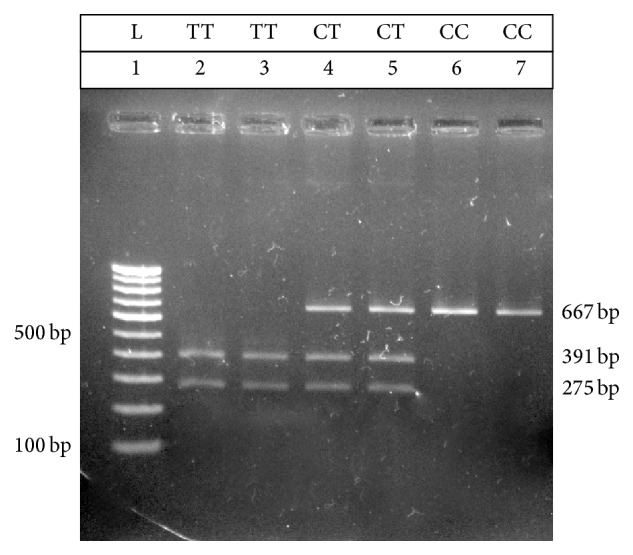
Gel representing typical restriction fragment length polymorphism patterns obtained by subjecting PCR products to restriction digestion by enzyme,* PagI* (New England Biolabs Inc., USA). Lane 1 consists of the 100 bp DNA marker (Bangalore Genei, India). Lanes 2 and 3 represent RFLP pattern for homozygous mutant alleles (TT). Lanes 4 and 5 represent RFLP pattern for heterozygous (CT) alleles. Lanes 6 and 7 represent RFLP pattern for homozygous wild type (CC) alleles.
